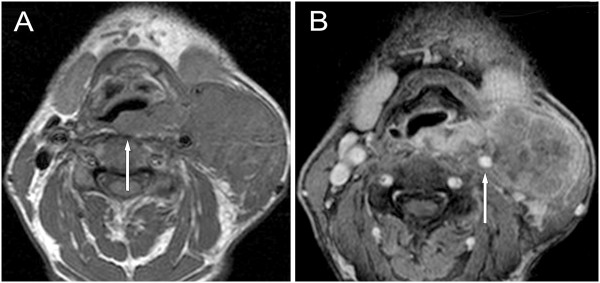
Hypopharyngeal cancer, stage T4bN3. A: transverse non-contrast T1-weighted, B: transverse post-contrast T1-weighted MR images with fat suppression demonstrate cancer of the dorsal hypopharynx wall passing through the retropharyngeal fascia disrupting the prevertebral fat plane (A image, arrow). A large 7 cm in diameter nodal conglomerate is visible on the left side, connected to the tumour. The primary tumour and the connected conglomerate encircles the internal carotid artery (B image, arrow), resulting an inoperable condition. The patient recieved radiochemotherapy.
